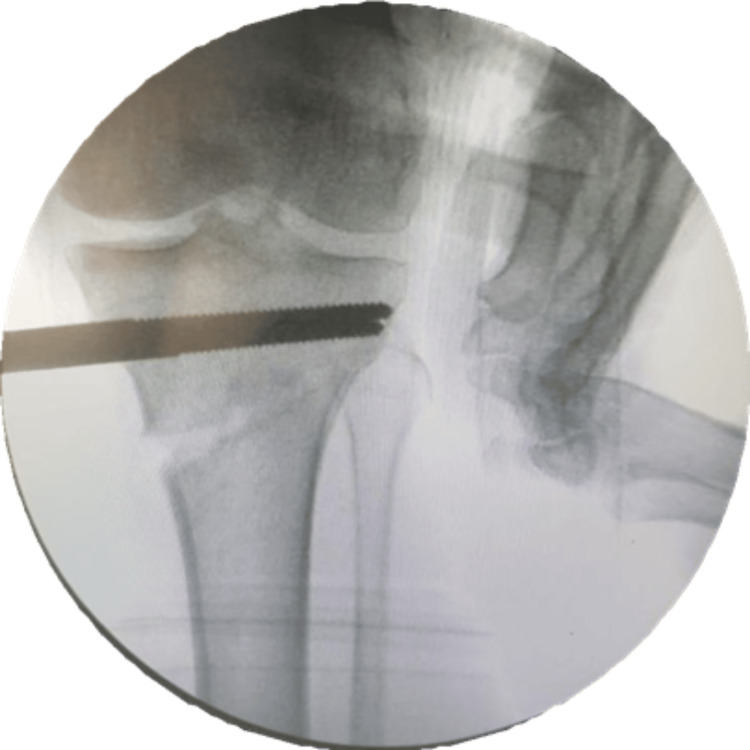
Radiograph image of medial open wedge osteotomy.
